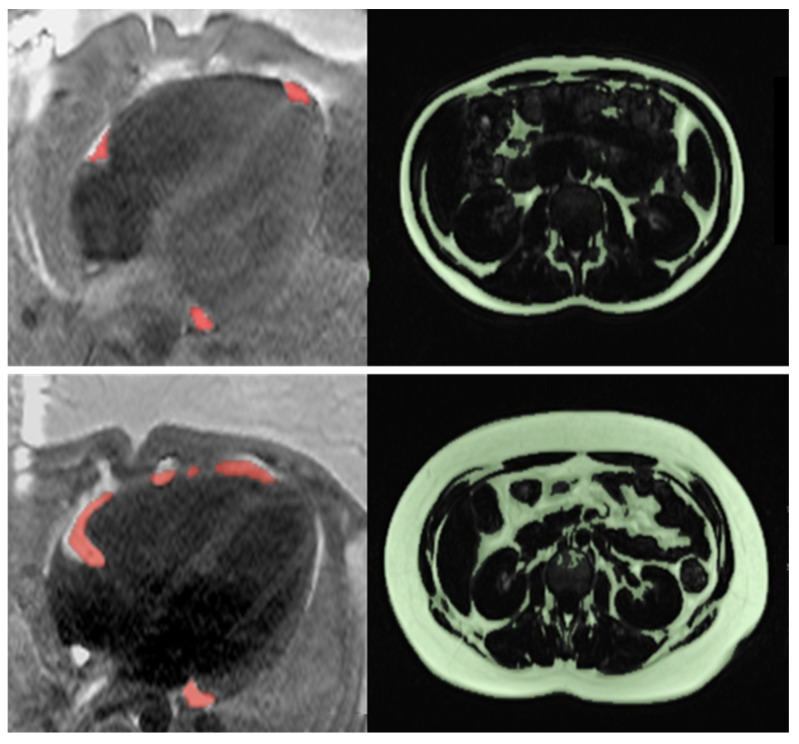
Representative examples of MRI epicardial and abdominal adipose tissue in a lean and an obese adolescent. (Top) Lean patient. Epicardial fat (in red) is located around the coronary arteries and at the apex. Total abdominal fat is delineated in green. (Bottom) Obese patient. Epicardial fat extends over the right ventricular border. Visceral and subcutaneous fat are significantly increased (green).
